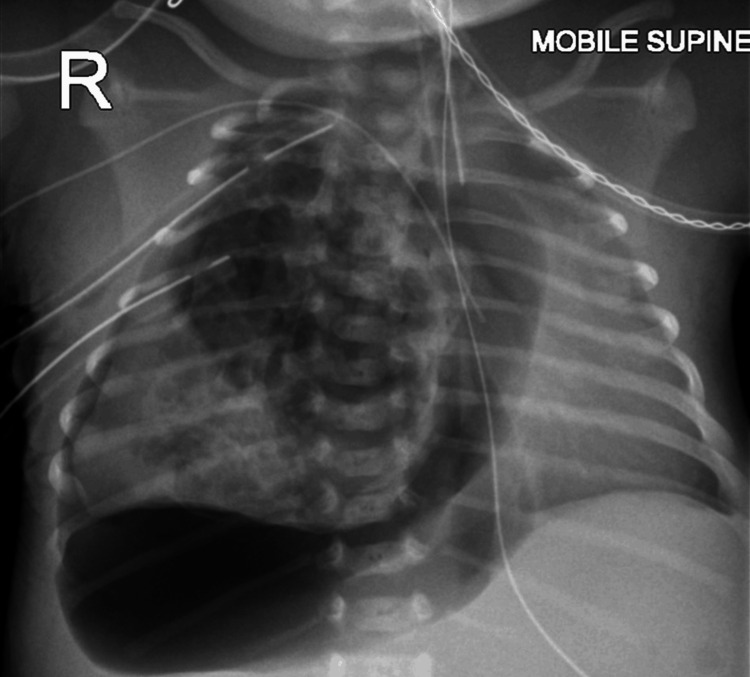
Chest X-ray of the newborn on day 1 of life showing a right-sided pneumothorax A large right tension pneumothorax with cystic lucencies in the collapsed right lung, consistent with the known CPAM. The left lung is partially aerated. CPAM, congenital pulmonary airway malformation
